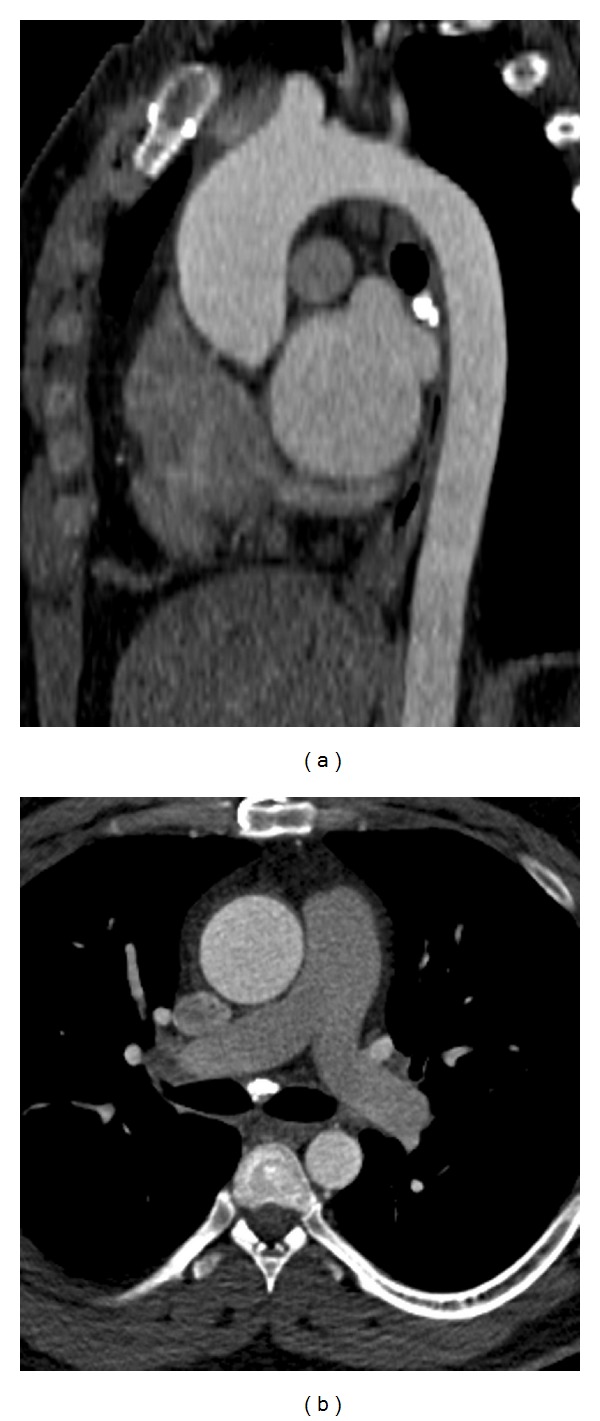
Aortic dilation—(a) sagittal oblique reconstructed CT in a 41-year-old male shows dilated ascending aorta (4.6 cm) not having reached the size required for a diagnosis of aneurysm. (b) Axial CT image shows the dilated ascending aorta but normal descending thoracic aorta.
